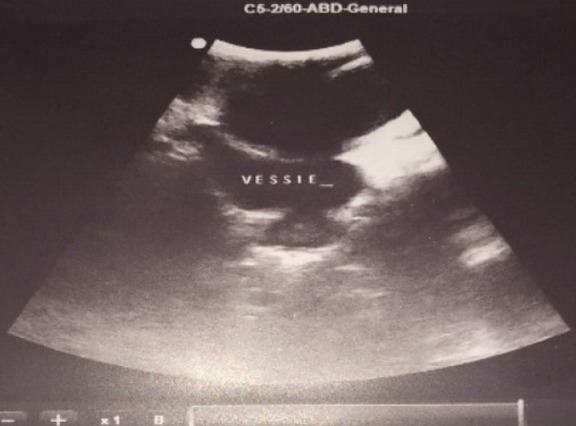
ultrasonographic imaging of the abdomen and pelvis (coronal section): intimate contact of the cyst with the bladder which is compressed
